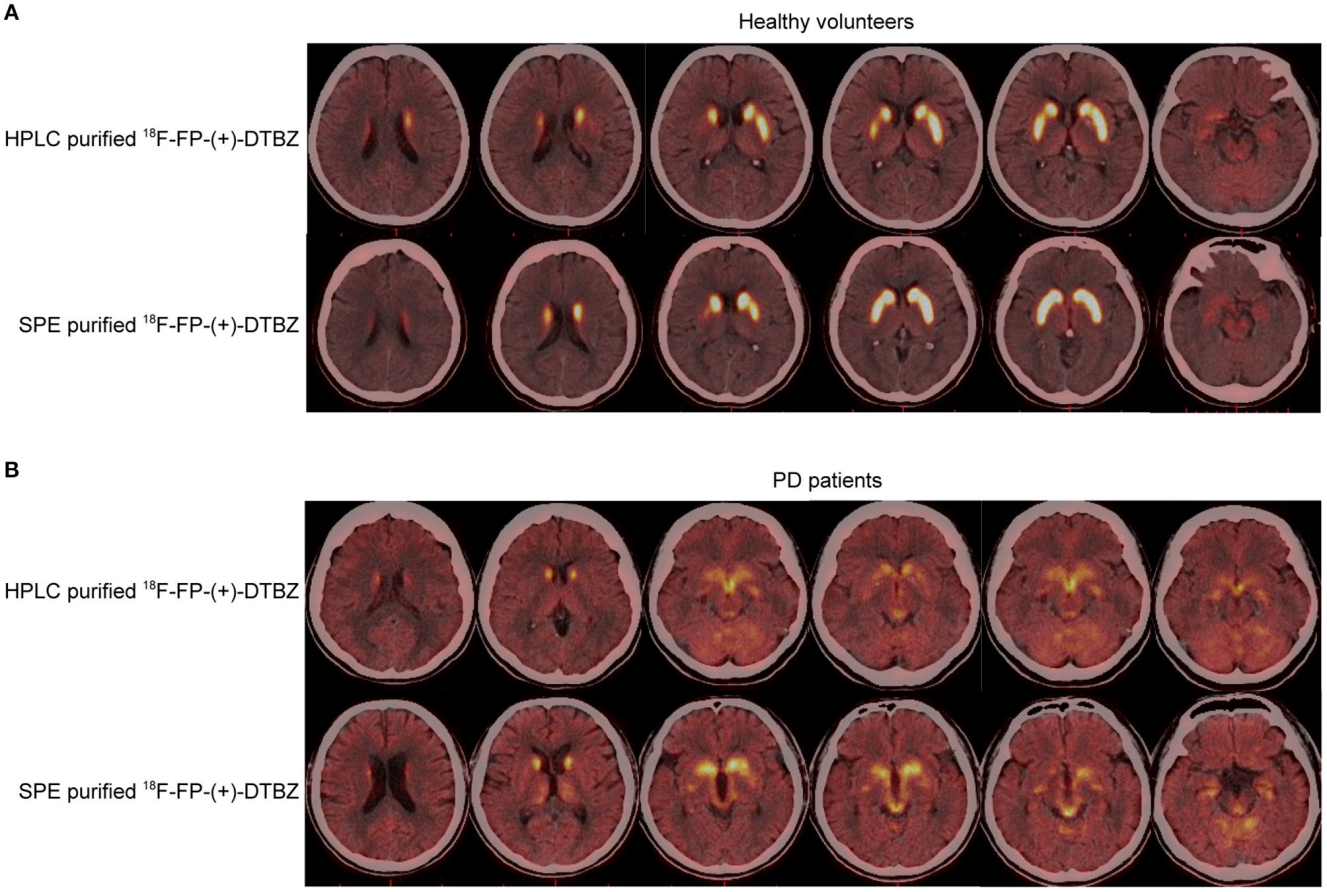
PET/CT performance of HPLC- and SPE- purified ^18^F-FP-(+)-DTBZ in healthy volunteers (A), and PD patients (B).
